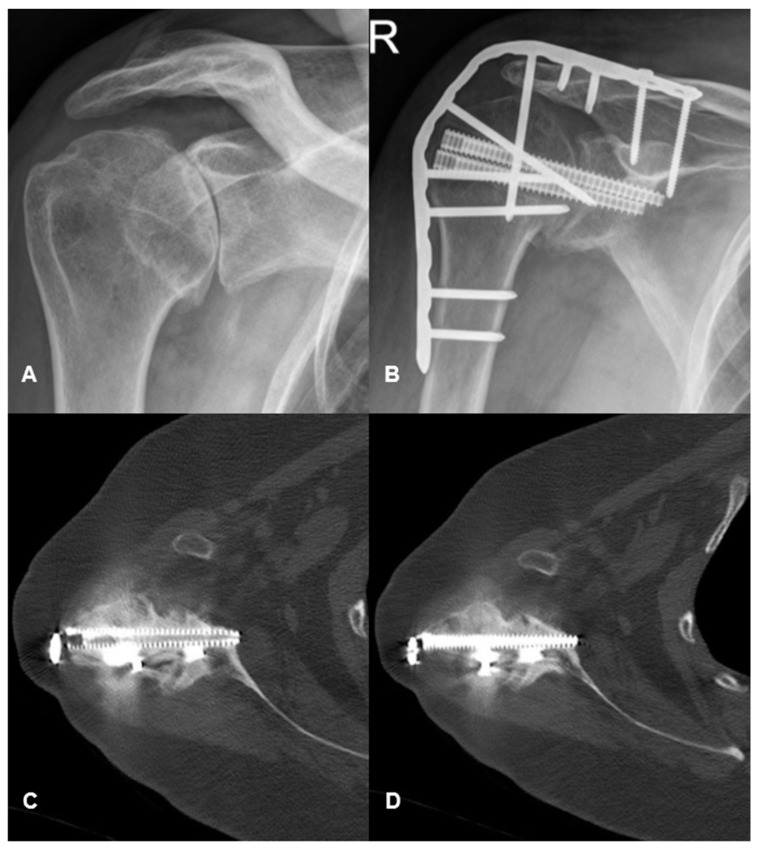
(A) shows an anteroposterior radiograph of the right shoulder demonstrating evidence of osteoarthritis in a patient with recurrent septic arthritis. (B) shows the same patient undergoing shoulder arthrodesis with screw and plate constructs. (C,D) are post-operative axial computed tomography scans demonstrating bridging callus and arthrodesis of the shoulder joint.
